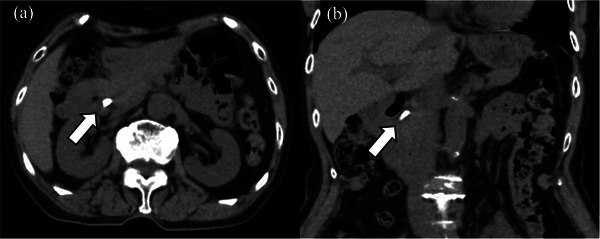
Non‐contrast computed tomography (CT) image((a) axial, (b) coronal) of the abdomen showed a hyperdense area (3137 Hounsfield units) in the distal bile duct.
